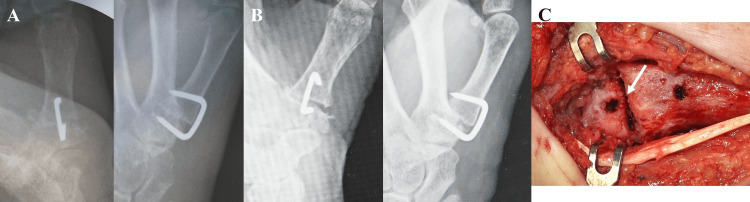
Use of a nitinol compression staple for arthrodesis of the thumb carpometacarpal (CMC) joint. (A) Intraoperative observation confirmed stable fixation with no gap at the fusion site. (B) Radiograph showing loosening at the fusion site after the patient reported pain following initiation of range-of-motion exercises. (C) Intraoperative photograph during revision surgery revealing a fracture around the staple leg (white arrow).
